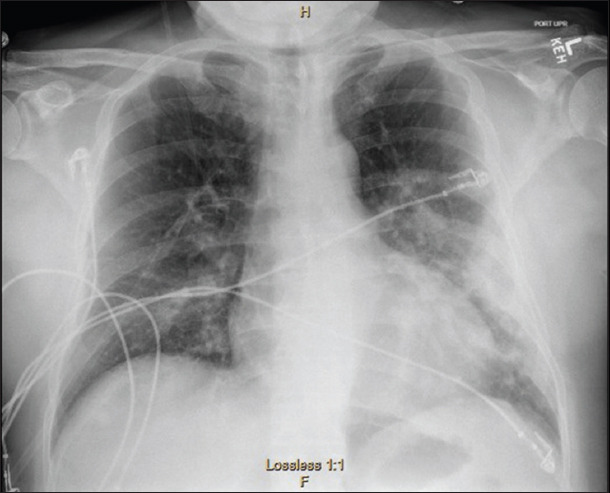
Chest radiograph showed unchanged airspace opacities within the left midlung zone. No pleural effusion or pneumothorax. Cardiomediastinal silhouette within normal limits. Pulmonary vasculature within normal limits. Unchanged osseous structures.
